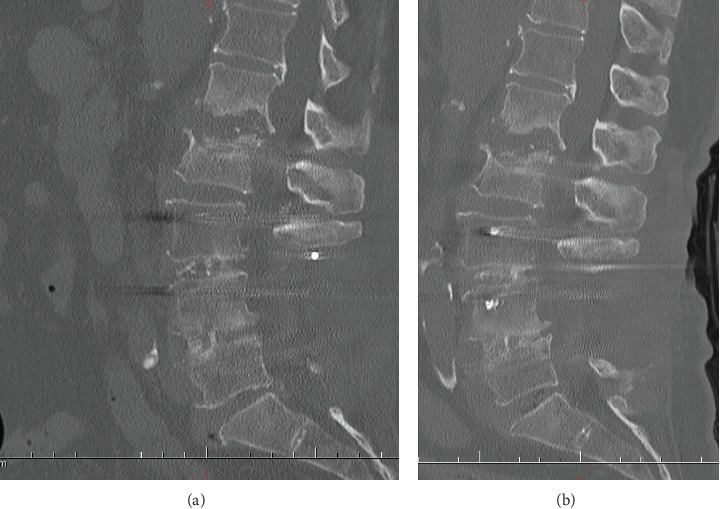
Sagittal lumbar CT after two-week anti-infective therapy (a). Sagittal lumbar CT after one-month anti-infective therapy showing little changes at the L1-2 space comparing with previous lumbar CTs during third hospitalization (b).
